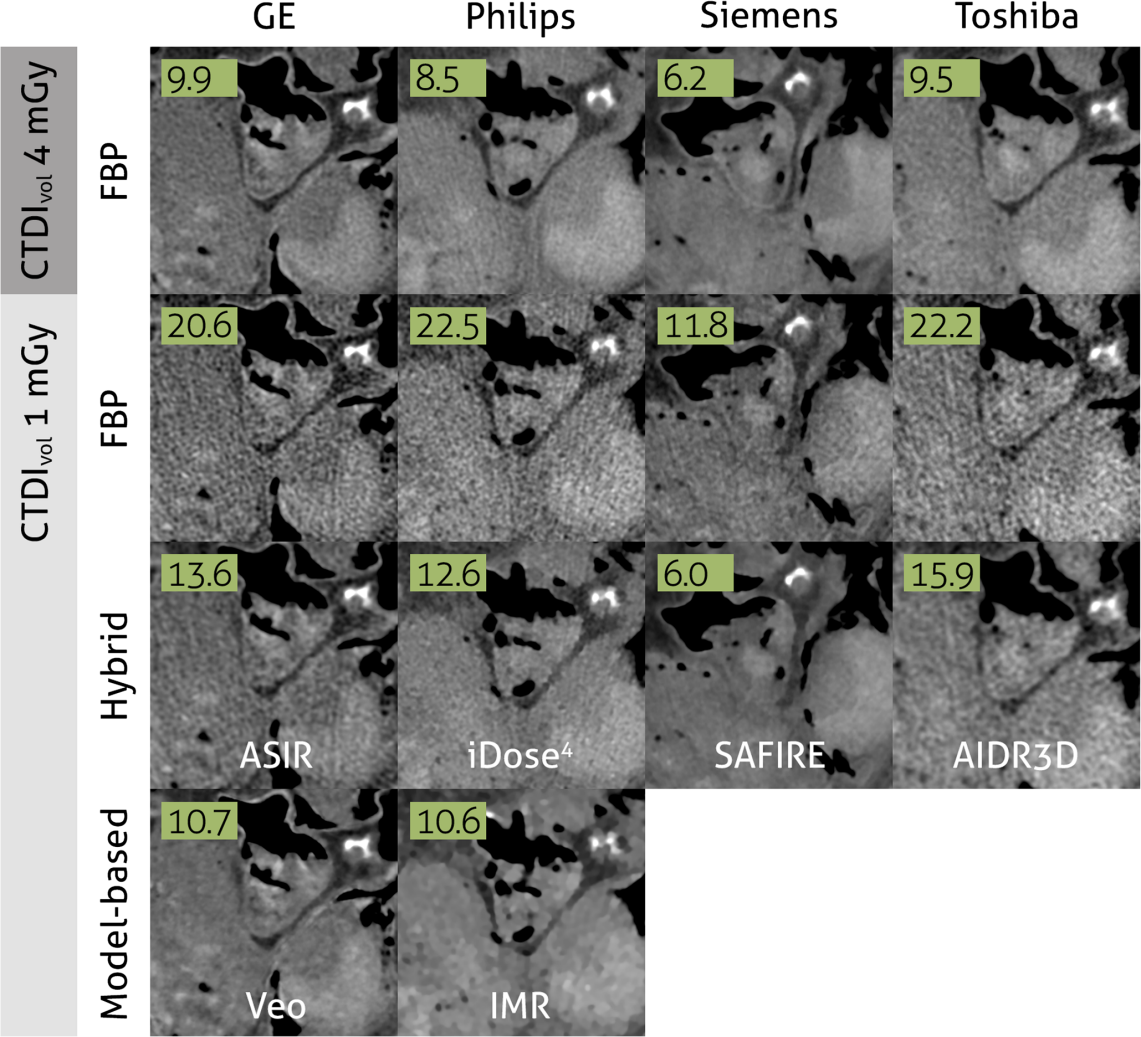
One ex vivo human heart, scanned at 4 mGy and 1 mGy (75% dose-reduction) with high-end CT scanners from four vendors. Images are reconstructed with filtered back projection (FBP), hybrid iterative reconstruction, and model-based iterative reconstruction. Numbers represent noise levels (standard deviations) in air. Images derived from a study published before by Willemink et al [39]
